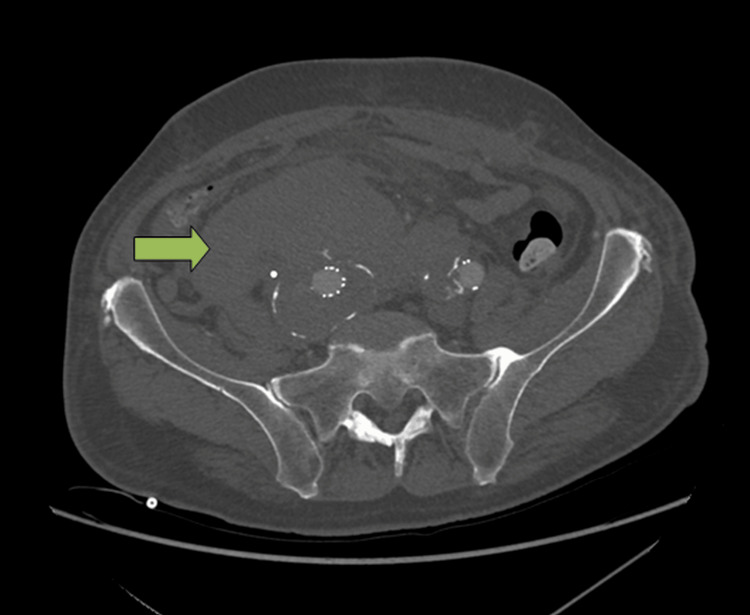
CT showed a large right-sided retroperitoneal hematoma (green arrow) extending from the level of the duodenum to the pelvis. The hematoma was adjacent to the aneurysm sac.
